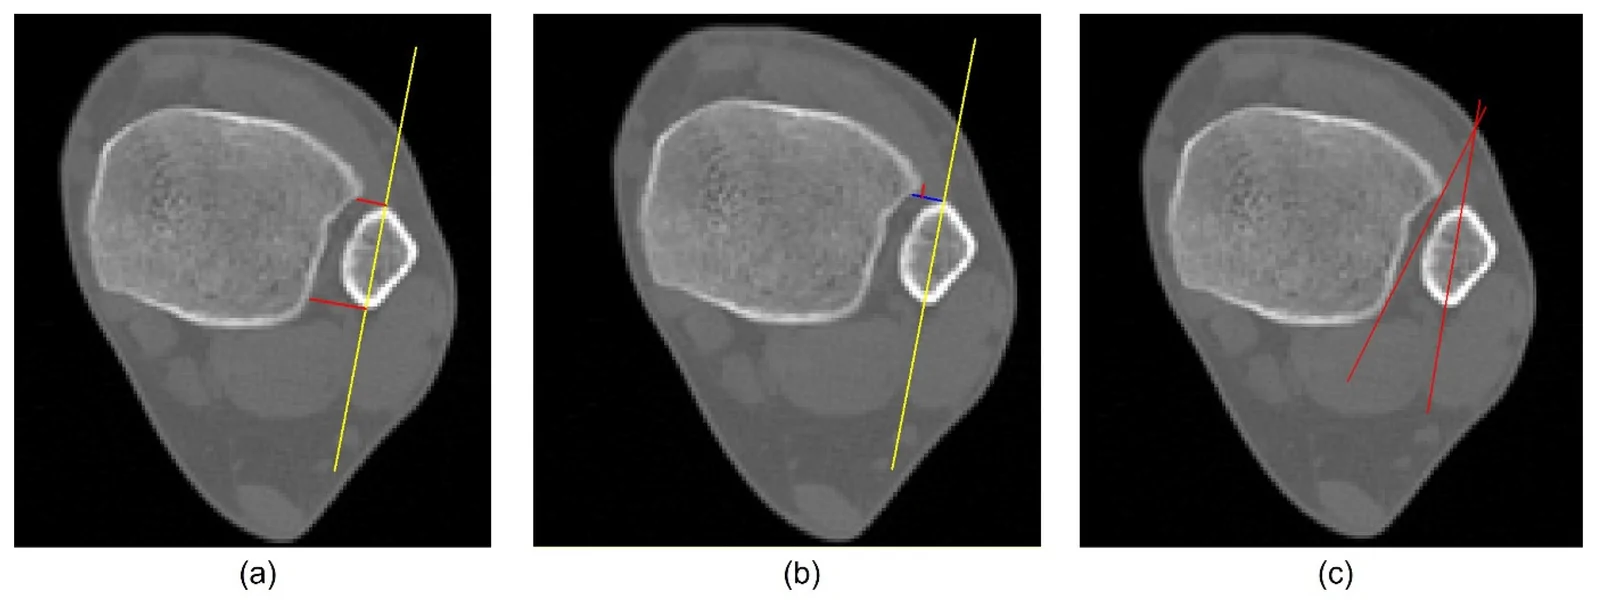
Computed tomography (CT)-based radiologic measurements used in this study. (a) Red lines: direct anterior difference (DAD) and direct posterior difference (DPD). Yellow line: fibular orientation line. (b) Red line: fibular translation. Yellow line: fibular orientation line. Blue line: DAD reference line, drawn through the anterior end of the fibular orientation line with the same orientation as the DAD measurement line. (c) Red line: fibular rotation.
